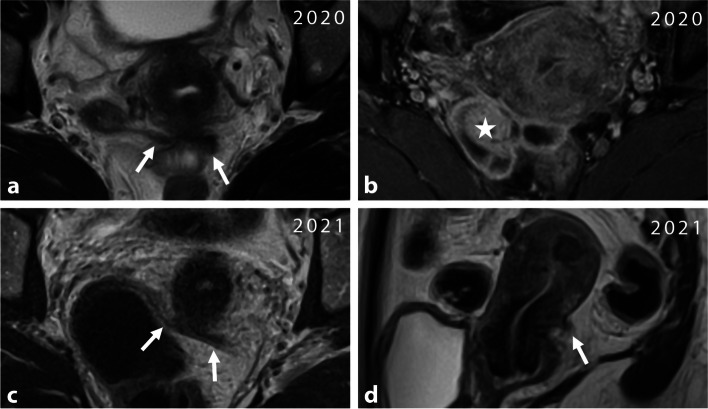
Thickening of the utero-sacral ligaments (USLs) in a 44-year-old woman with history of PID. *Acute episode in 2020:*a Axial T2-W MR image shows irregular and pseudo-nodular thickening of bilateral USLs (arrows). b Axial T1-W fat-suppressed contrast-enhanced MR image shows pyosalpinx (star) with thick-walled fallopian tube and surrounding fat stranding. *One year follow-up in 2021:*c Axial and (d) sagittal T2-W MR images show persistence of pseudonodular thickening of the utero-sacral ligaments (arrows) and the torus, without hemorrhagic foci (not shown)
